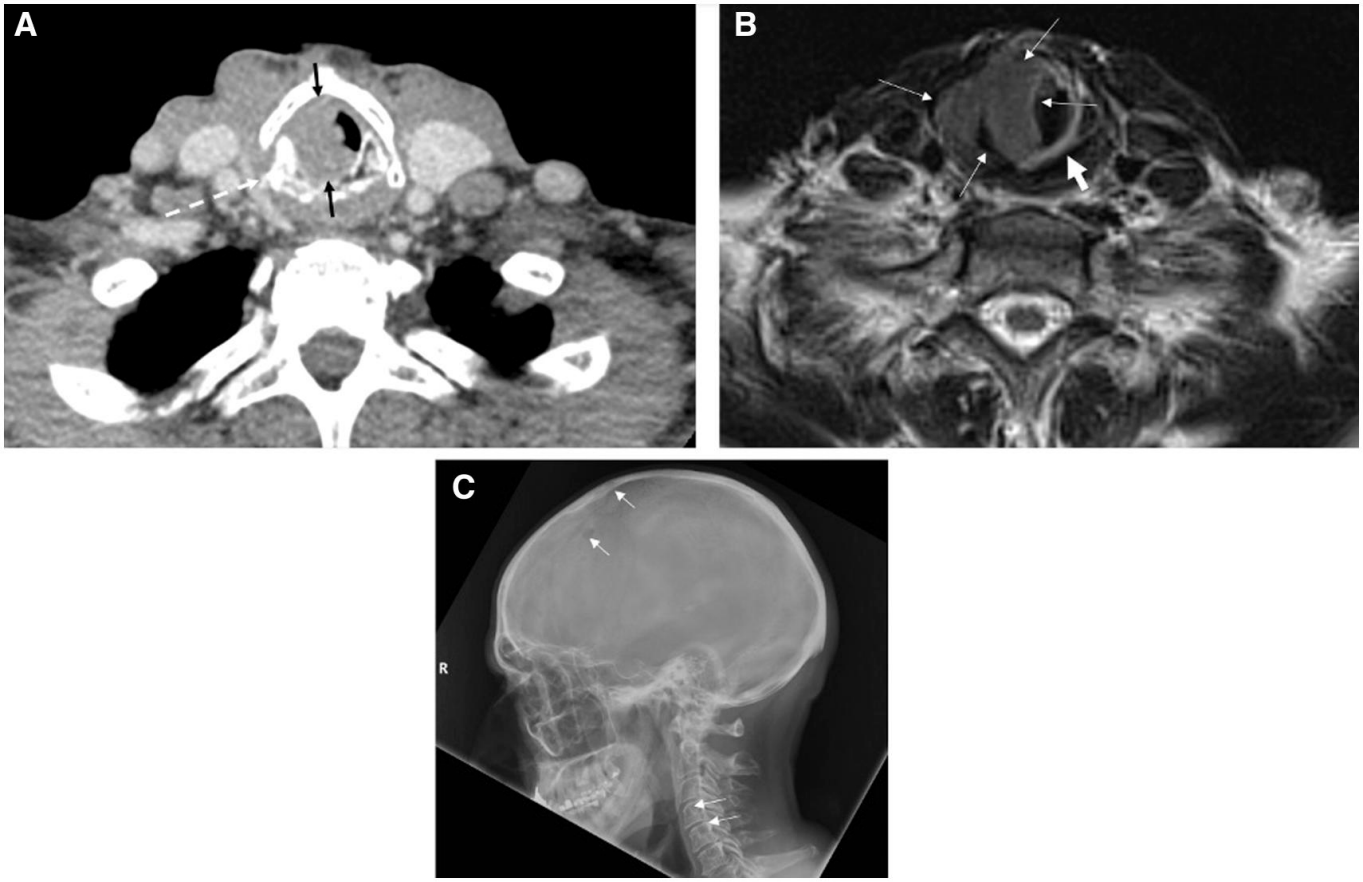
Subglottic and right cricoid ring EMP in MM relapse. (A) Axial CECT shows a homogeneous lobulated mass (thin black arrows) that almost occludes the subglottic larynx. The right cricoid cartilage is sclerotic (dotted arrow). (B) Axial T2-weighted MRI shows homogeneous intermediate signal mass. Note the high T2-weighed signal differentiating tumour (thin white arrow) from normal adjacent laryngeal mucosa (short white arrow). (C) Sagittal radiograph of the calvarium from a historic MM skeletal survey in the same patient as Figure 2A and B demonstrates multiple lytic skull vault and C4-6 vertebral lesions (short thin white arrow).
